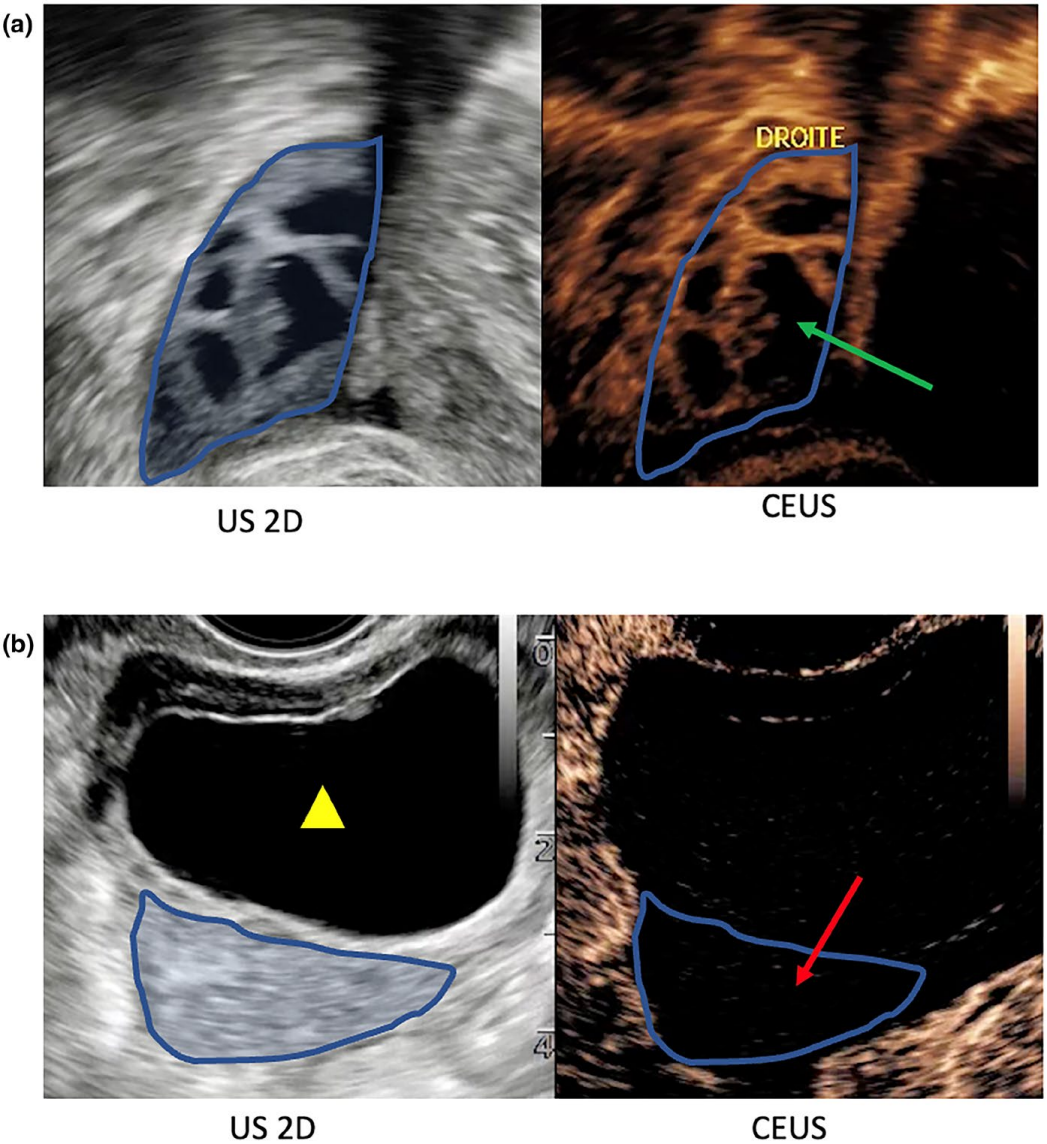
Contrast‐enhanced ultrasound in a control case (a) and ovarian torsion case (b). Transvaginal ultrasound in axial plan in two‐dimensional (2D) and contrast mode. In the 2D ultrasound image (US) (on the left), the ovarian parenchyma is outlined in blue, and the ovarian cyst is outlined by a yellow triangle. In the contrast image (CEUS) (on the right), we can see enhancement (green arrow) or lack of enhancement of the ovarian parenchyma (red arrow).
